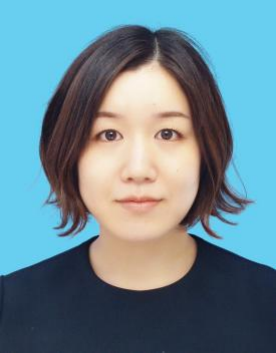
Kanna Nakamura was born in Osaka, Japan, in 1994. She received an MD degree from Osaka Medical and Pharmaceutical University, Osaka, Japan, in 2019. Her main areas of interest are echocardiography, cardiac magnetic resonance imaging, heart failure, and cardio-oncology.
